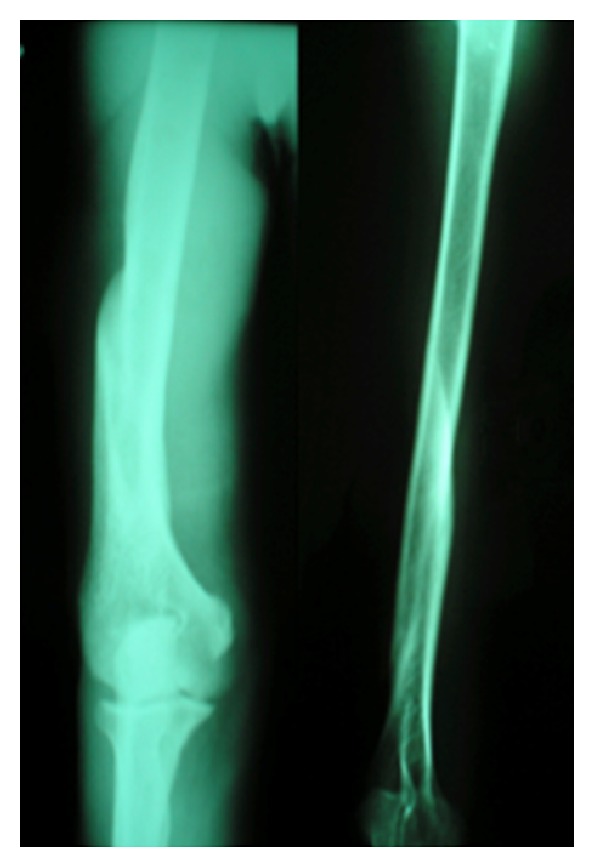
X-rays after brace removal.
